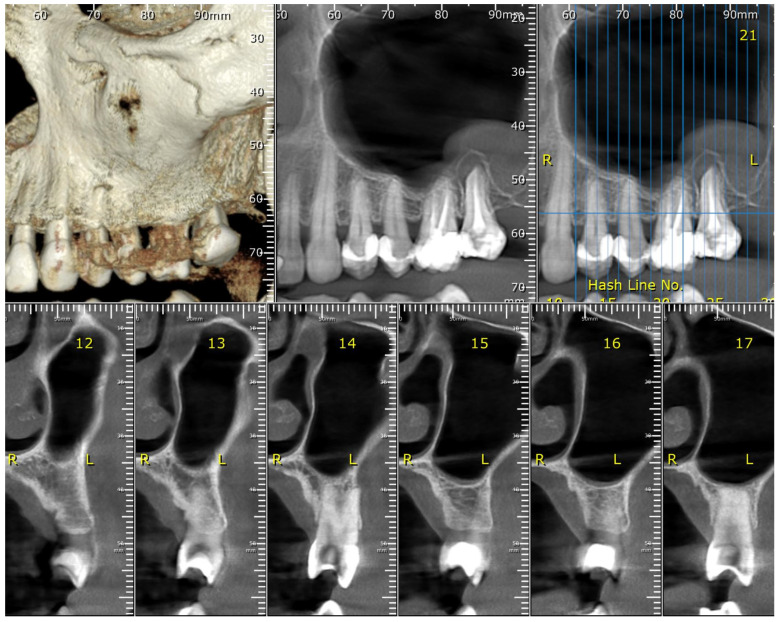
The maxillary CBCT scan reveals levels of bone loss, both vertical and horizontal.
